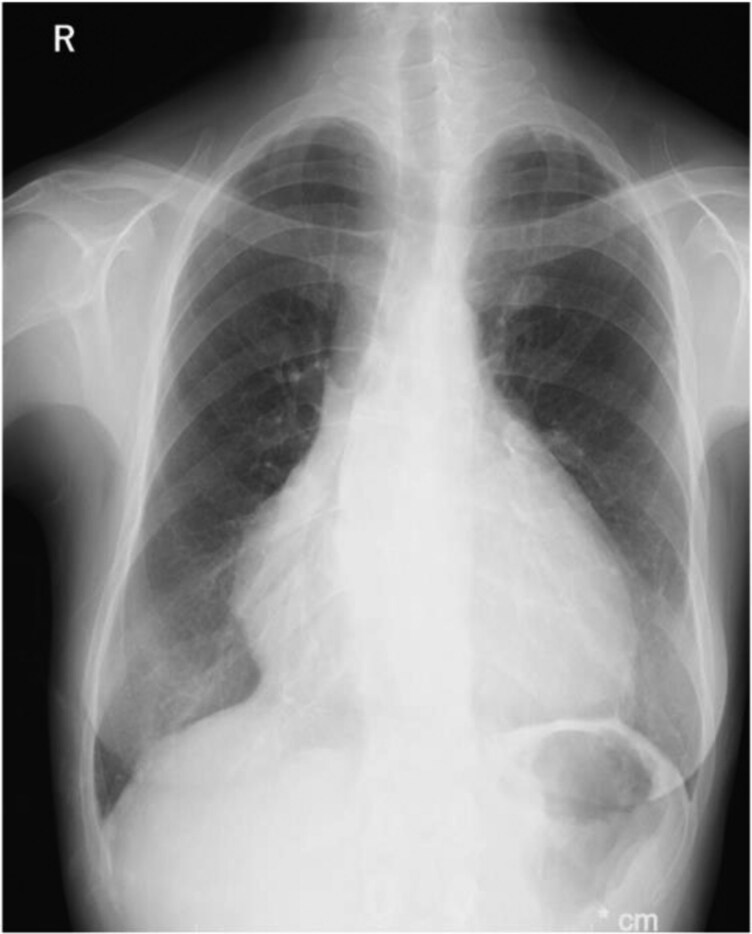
Chest radiograph showing cardiomegaly with a cardiothoracic ratio of 63.5%.
